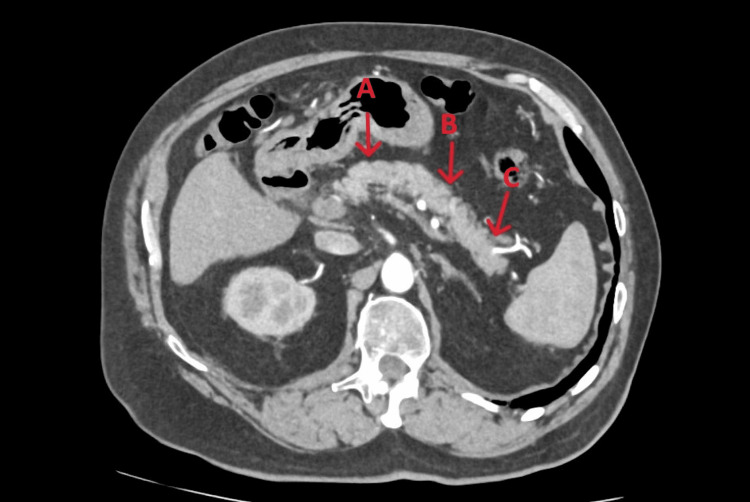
Computed Tomography (CT) pancreas showing normal tail/body without any pathology Arrows A and B indicate the body, and arrow C indicates the tail.
